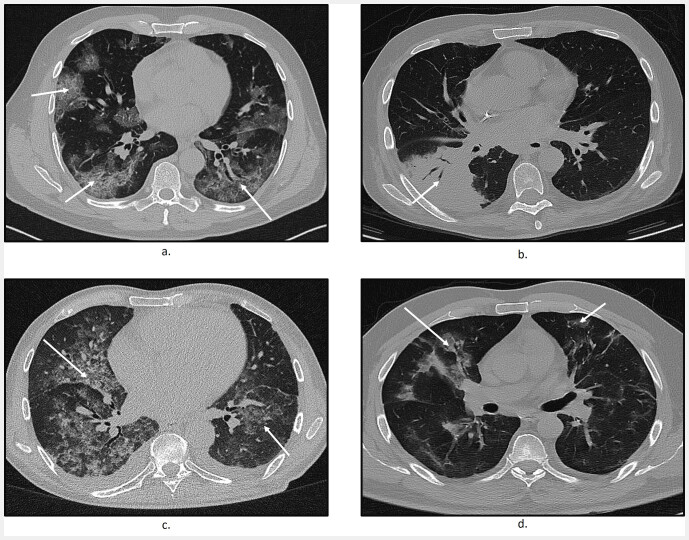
Slices from CT scans of nCP (viral pneumonia, bacterial pneumonia) and COVID-19 patients as well as predictions from the three diagnostic models.
